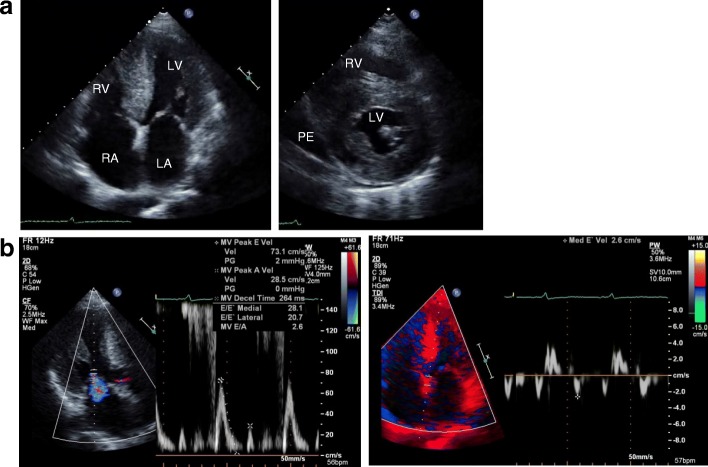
Transthoracic echocardiography in apical four chamber (a, *left panel*) and short axis view (*right*). Transmitral flow revealed an early mitral inflow to late filling velocity ratio of 2.6 (b, *left panel*). Tissue Doppler imaging of the mitral annulus (*right*). *LA* left atrium, *LV* left ventricle, *PE* pericardial effusion, *RA* right atrium, *RV* right ventricle
